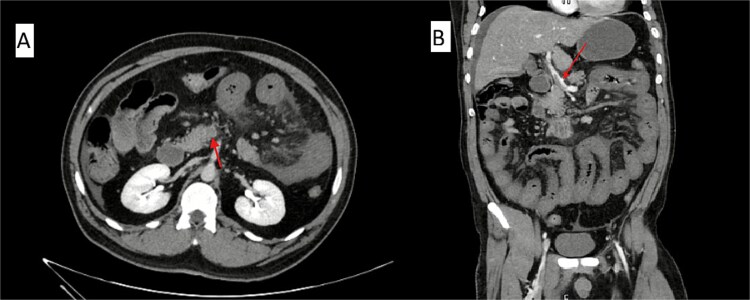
Contrast-enhanced abdominal CT scan showing acute mesenteric venous thrombosis. (A) Axial view demonstrating complete thrombosis of the superior mesenteric vein (arrow). (B) Coronal view confirming thrombus extension into the portal venous system (arrow).
